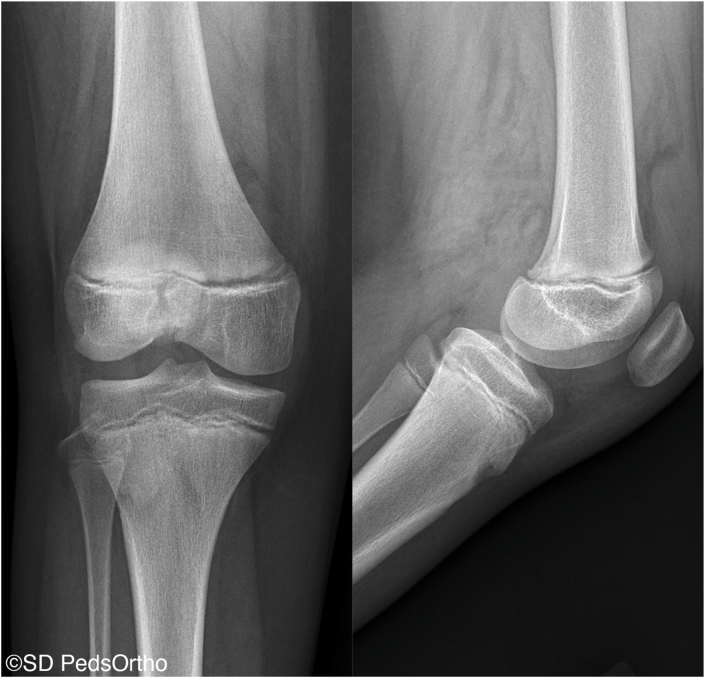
Radiograph of a right knee injury in a 10-year-old female.
